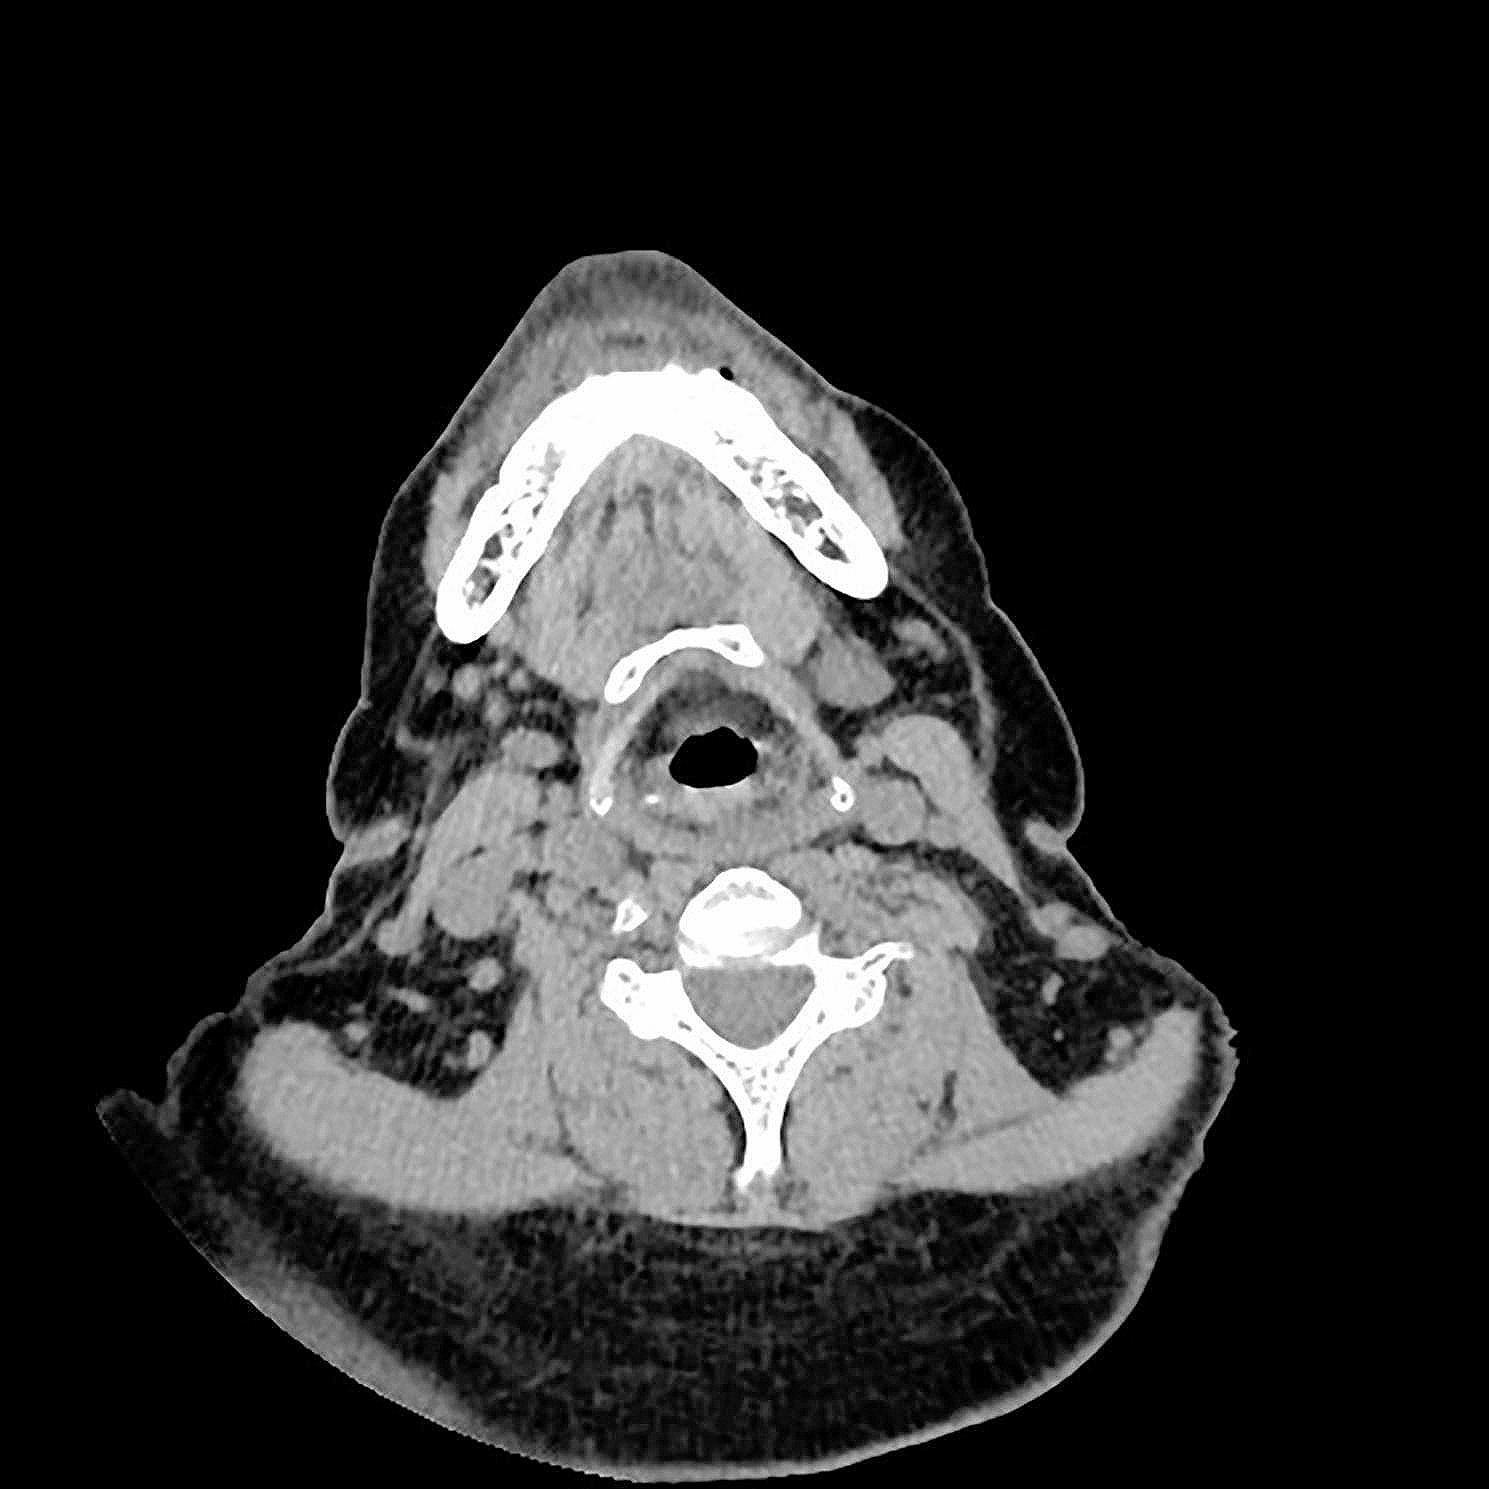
Neck mass evaluation through CT imaging
